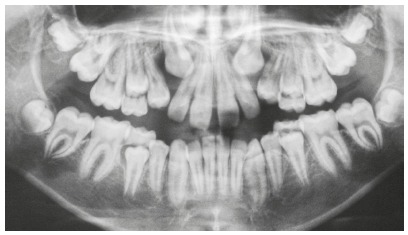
Panoramic radiograph shows displacement of permanent maxillary lateral incisors and first premolars due to impaction of #13 and #23.
